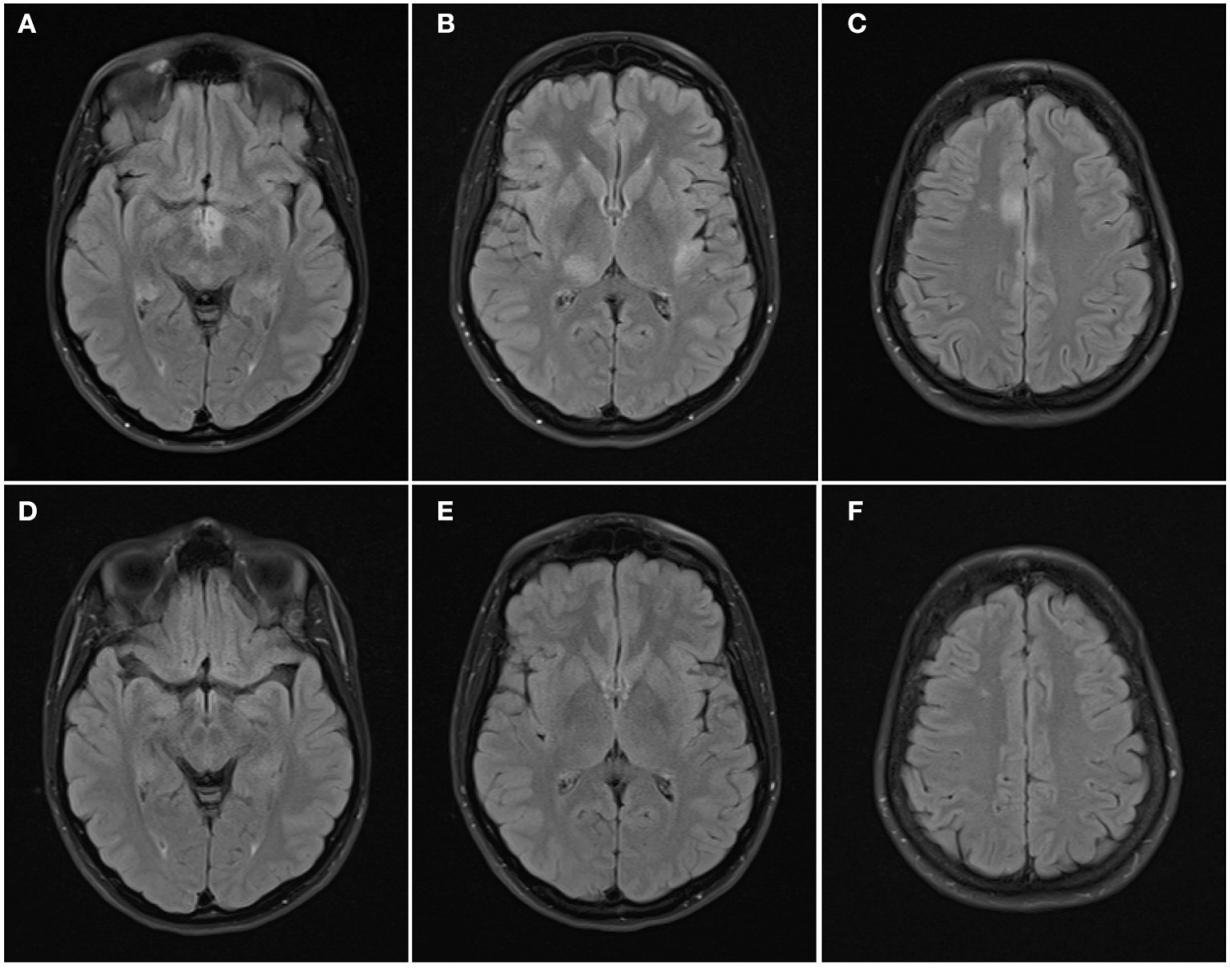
Axial FLAIR images of the brain from the same patient across serial examinations. (A–C) At the time of disease presentation: FLAIR hyperintense lesions involve the hypothalamus and optic tracts (A), right thalamus and left insular cortex (B), and parasagittal frontal cortex bilaterally and right centrum semiovale (C). (D–F) 5 months later: the lesions have all resolved except for the lesion in the right centrum semiovale.
